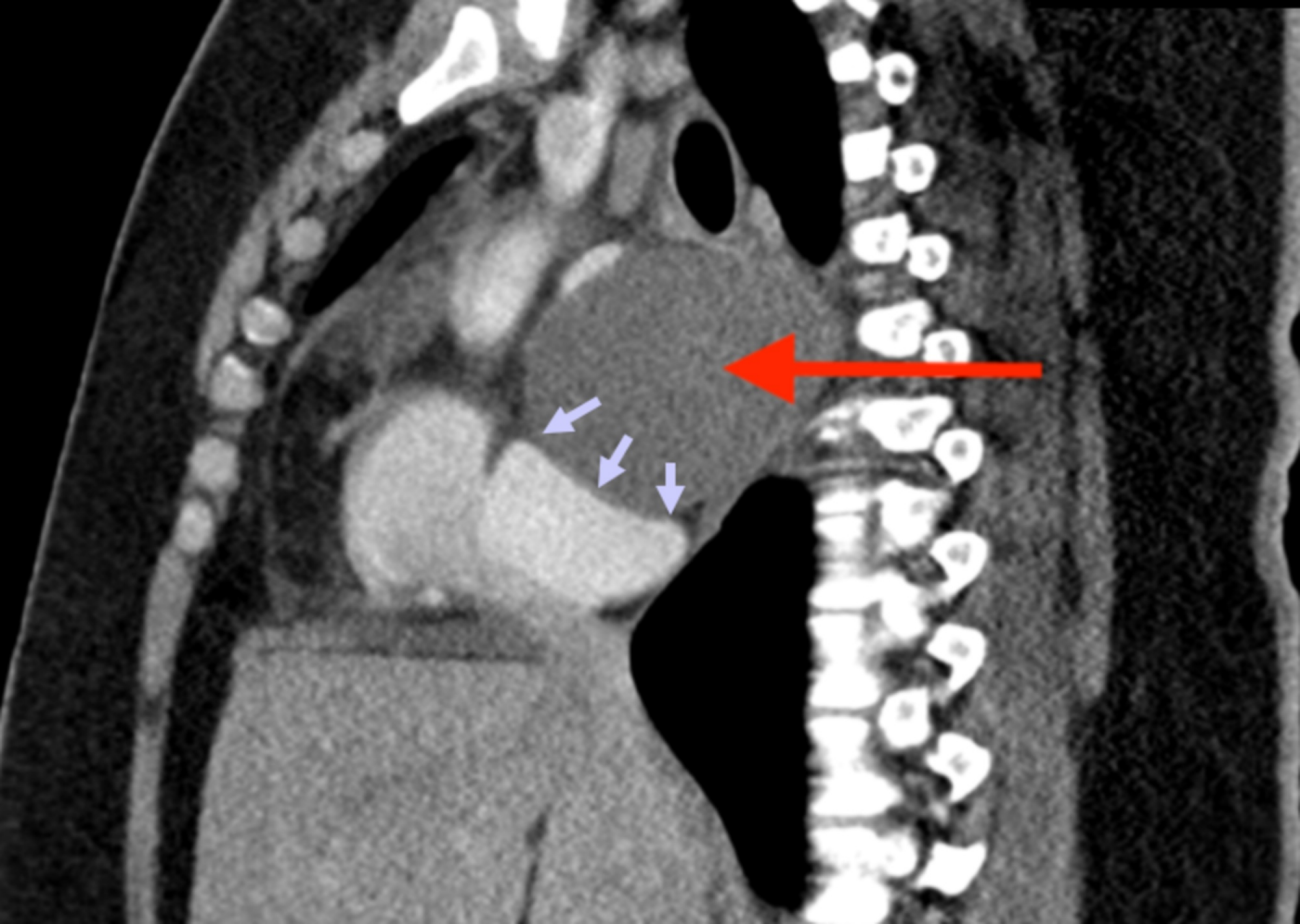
Chest CT (sagittal view) with intravenous contrast showing fluid-filled subcarinal mass (red arrow), causing a mass effect upon the left ventricle (purple arrows).
